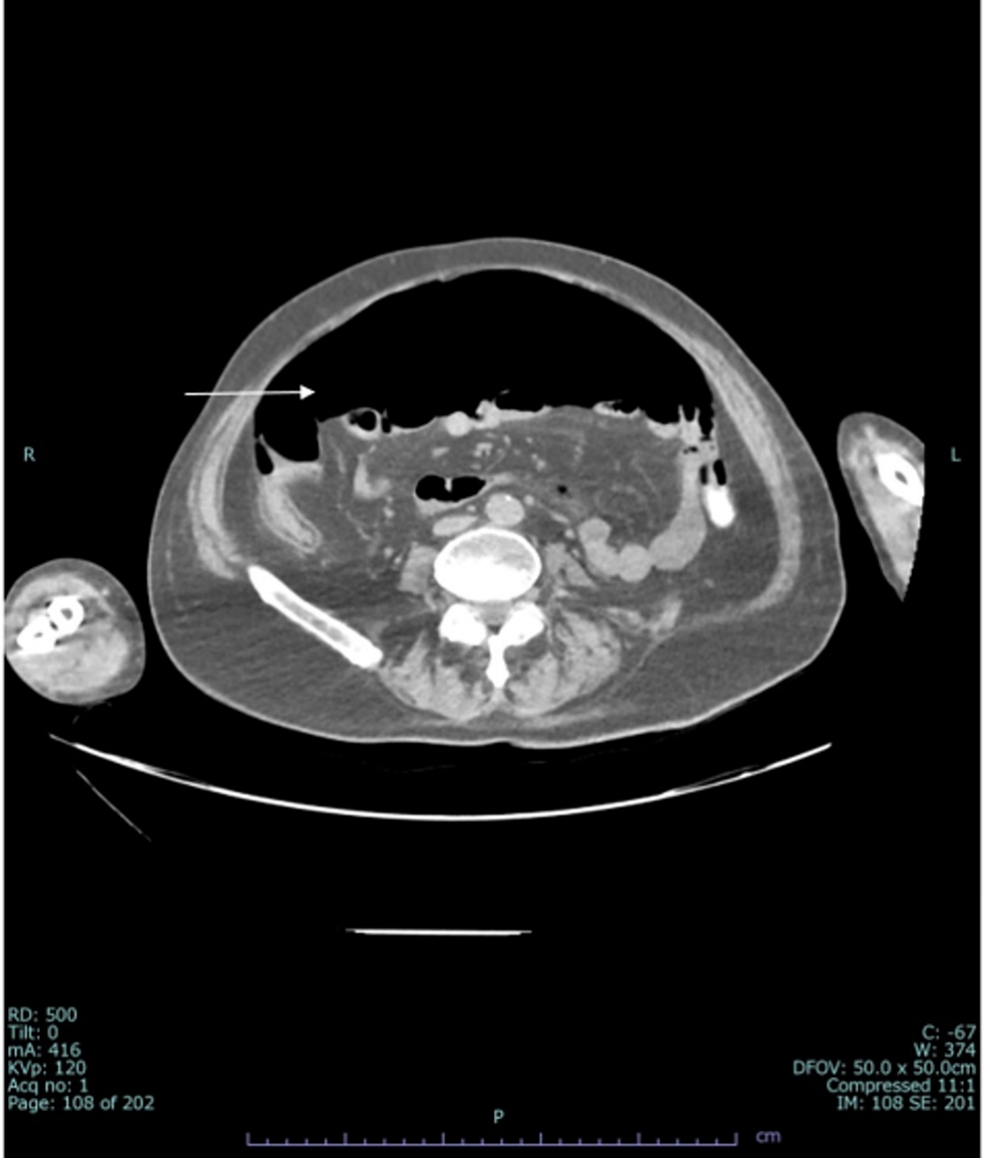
Axial CT of the abdomen showing large pneumoperitoneum (arrow) CT: computed tomography
